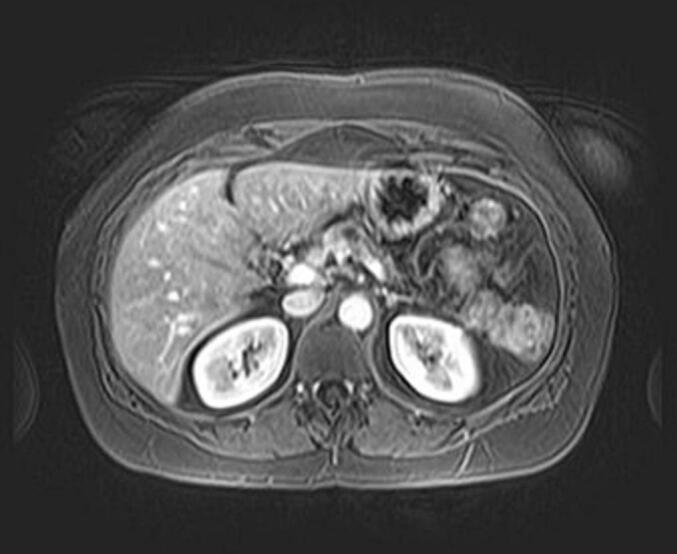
Magnetic resonance imaging (MRI) showing a cystic lesion in the body of the pancreas, in close contact with the splenic vessels.
